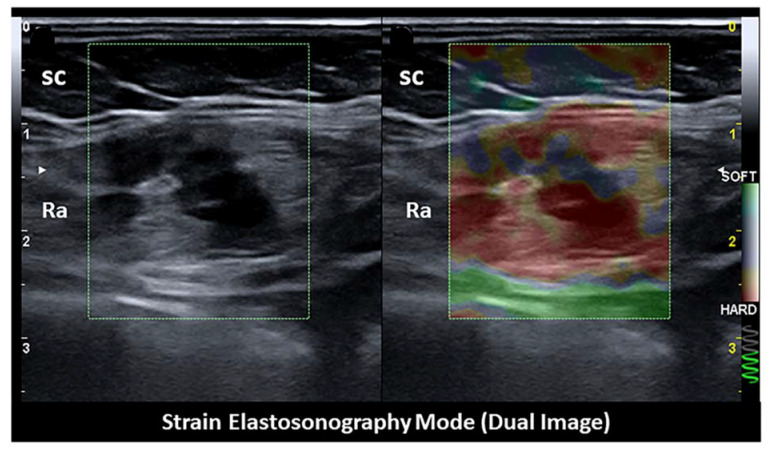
Strain elastosonography mode revealing the hard pattern of the endometriotic nodule. sc: subcutaneous tissue. ra: rectus abdominis muscle.
